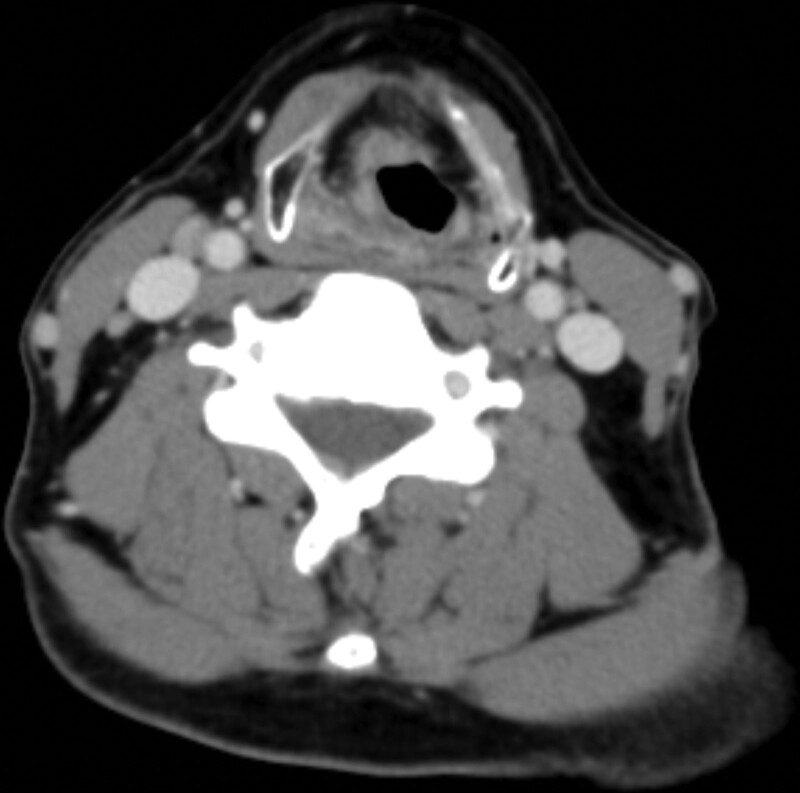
Tumor was seen over the right hypopharynx on CT scan. CT = computed tomography.
